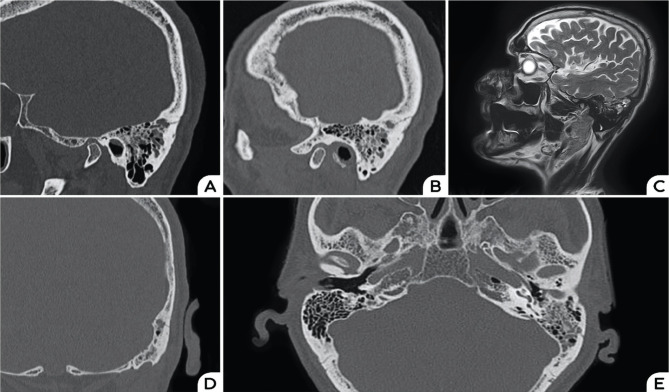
Sagittal hr-CT reconstruction of the temporal bone showing bone fracture involving the mastoid cells (A) and (B); Sagittal T2- weighted MRI showing hyperintense signal in the left mastoid cells (C); hr-CT scan reconstruction in coronal and axial planes showing the fracture involving an antral cell (D) and partial opacification of the left mastoid cells (E).
